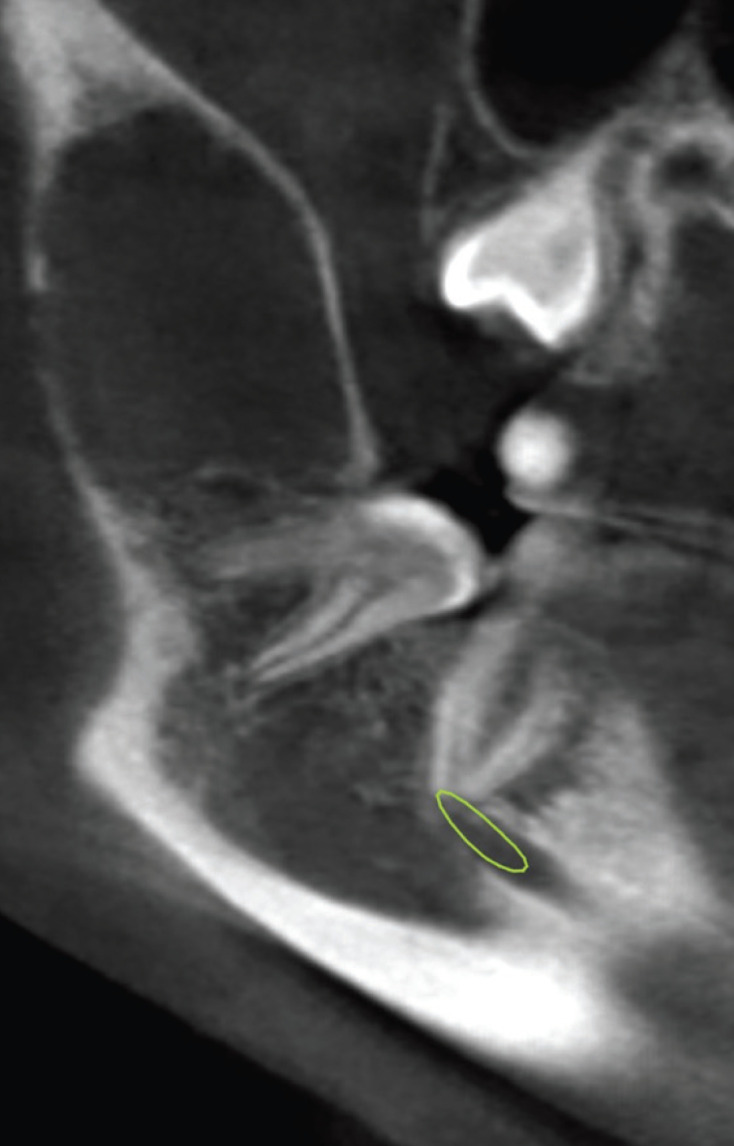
Cone-beam computed tomography of the simple bone cyst. Sagittal view.
